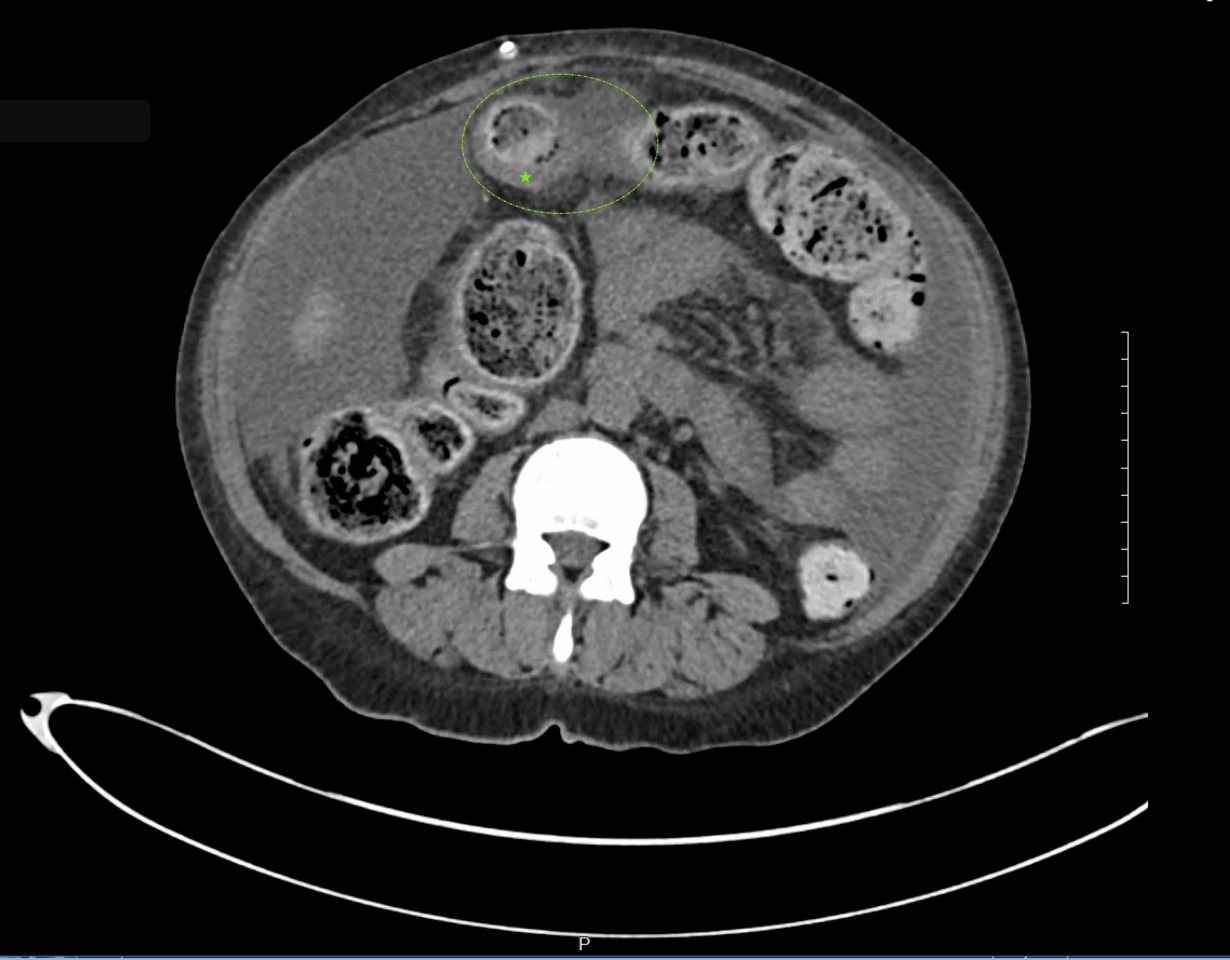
CT imaging of the abdomen revealed several findings including constipation, which can be seen within the dashed green circle. The green star indicates an area of bowel wall thickening CT: computed tomography
